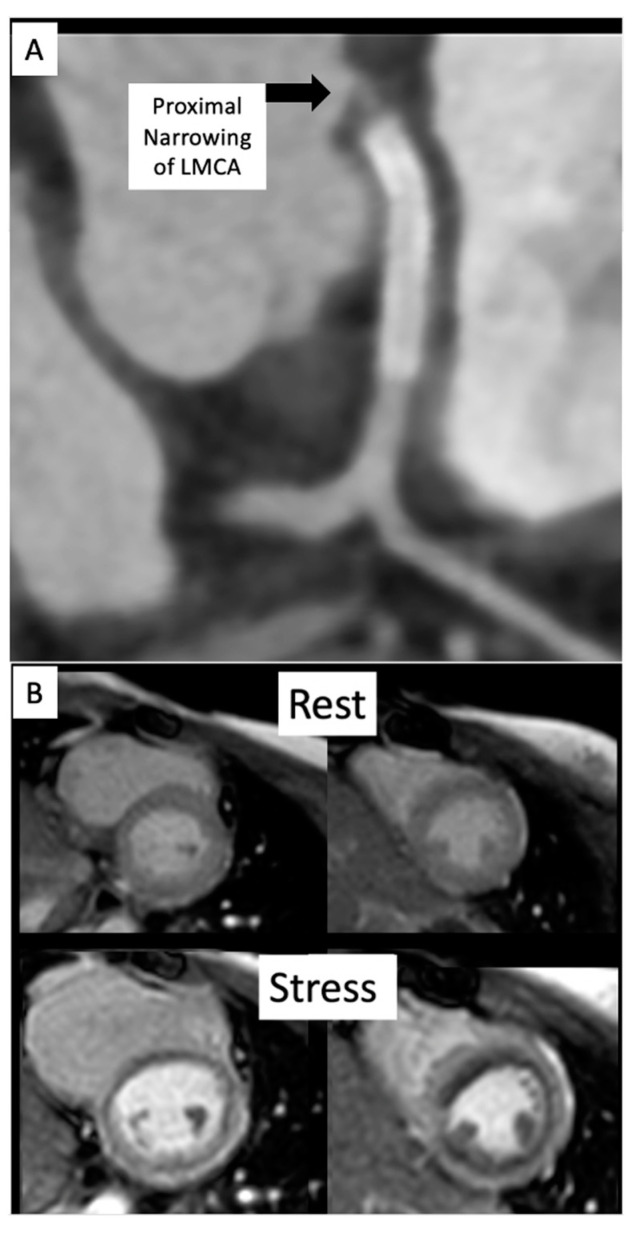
(A) CT on follow-up for patient from Figure 20 demonstrates a segment of near complete occlusion at the most proximal aspect of the left main coronary artery (LMCA), immediately prior to the previously placed stent. (B) Cardiac MRI demonstrated inducible perfusion defects along the anteroseptal, anterior, anterolateral, and lateral walls, at the basal and mid-ventricular level, as well as in the anterolateral papillary muscle (territory of the LMCA). No areas of late gadolinium enhancement to indicate myocardial fibrosis/injury are present. Patient proceeded to the catheterization lab for additional stenting of the LMCA ostium.
